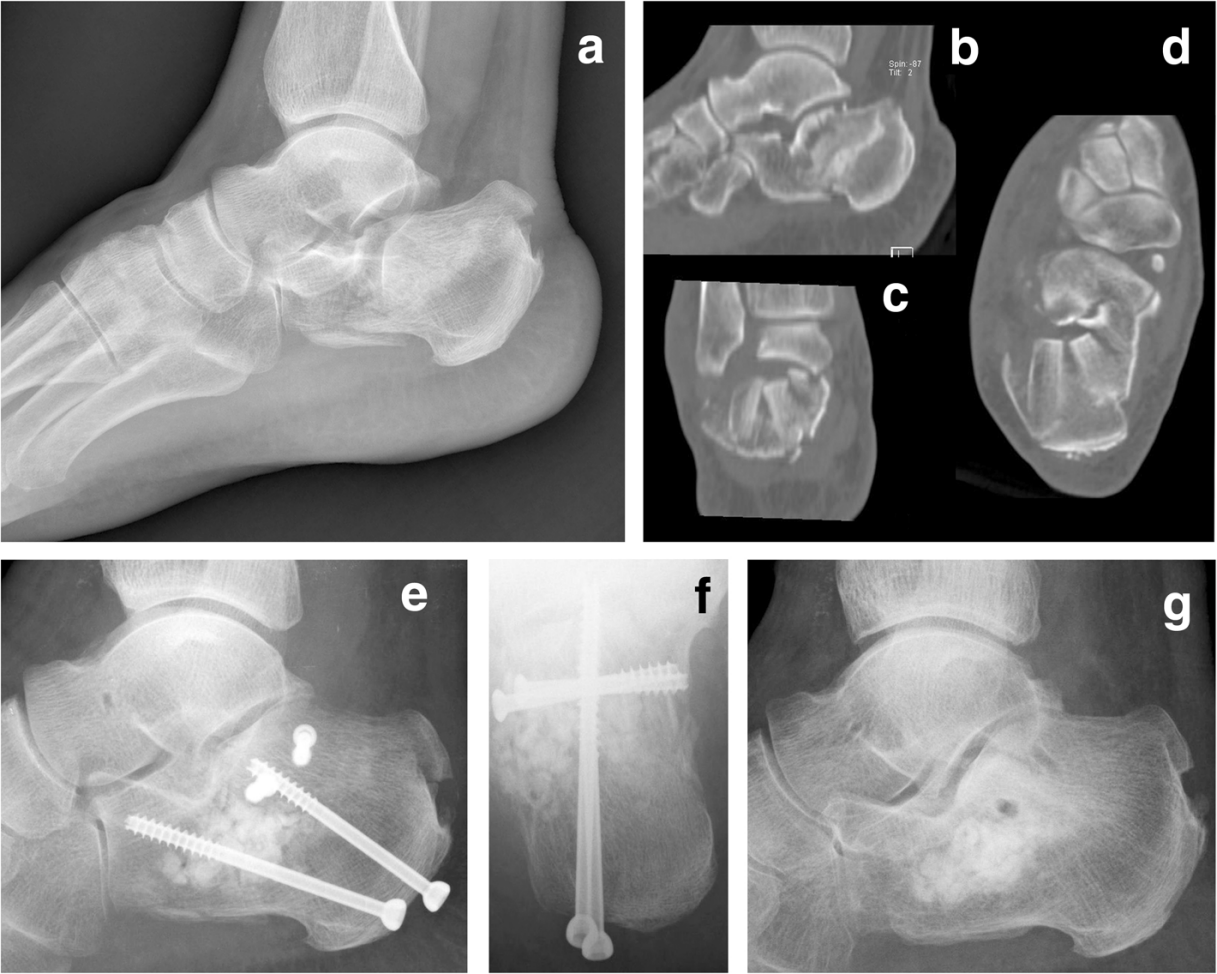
Male 62 years old: (a–d) preoperative X-ray and CT; (e–f) 30 days post op.; (g) follow-up 1 year later
